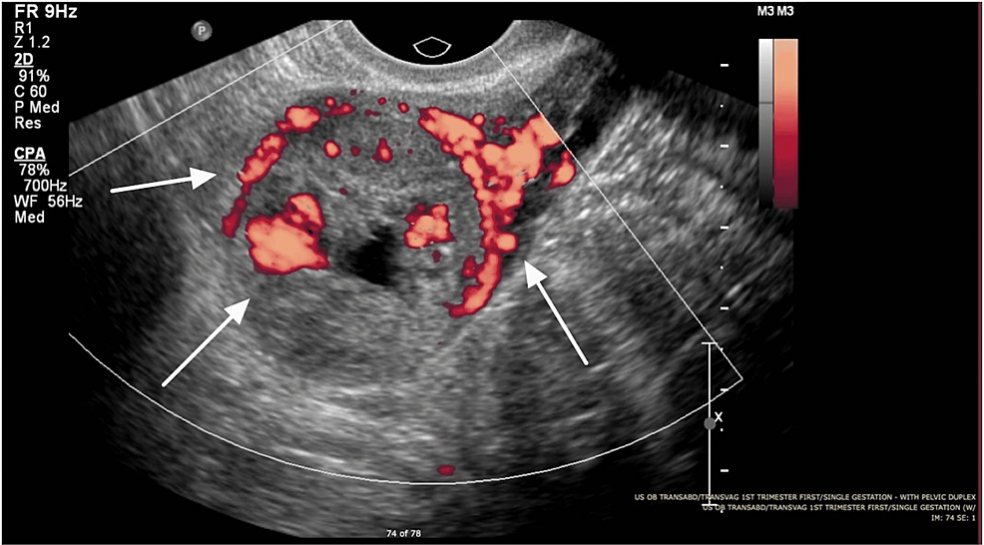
Doppler ultrasound of interstitial ectopic pregnancy. There is peripheral and internal flow on color Doppler indicated by the white arrows in a characteristic “ring of fire pattern.”
